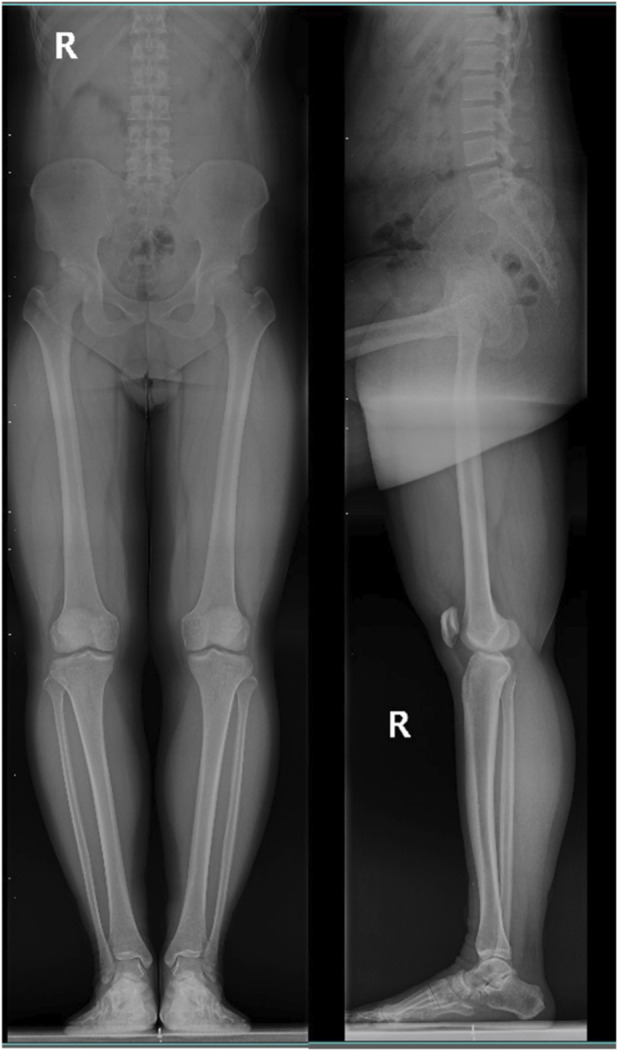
Radiographic projection.
